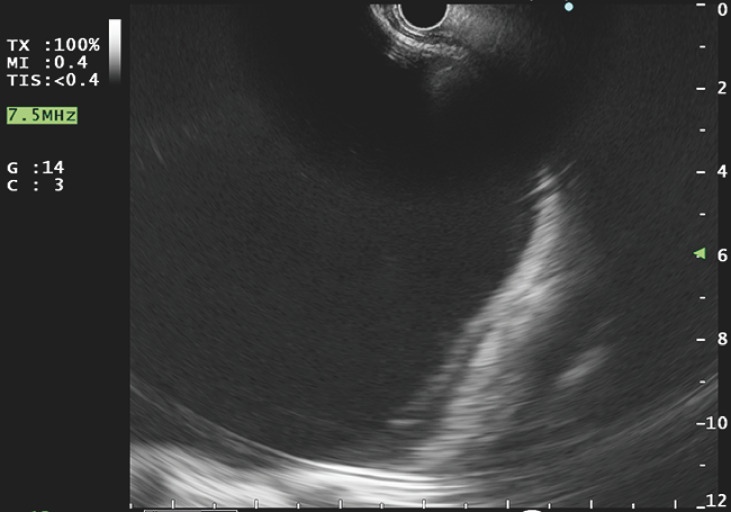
Endoscopic ultrasound image of a large anechoic cystic collection with a left ventriculoperitoneal shunt entering it.
